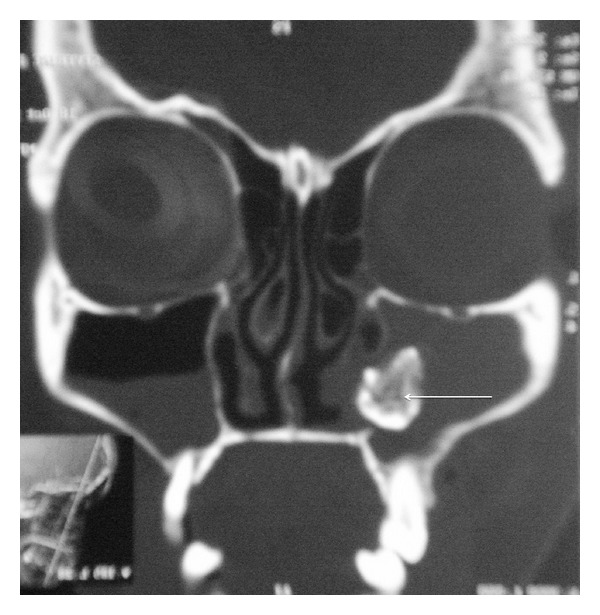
Coronal CT OMC showing antrolith.
